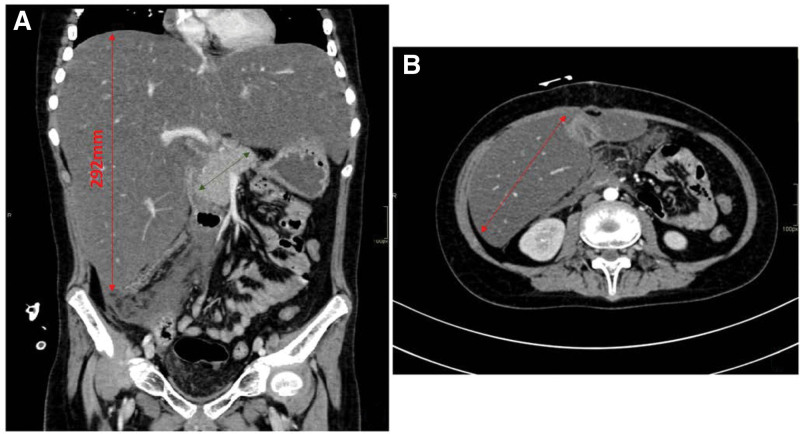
(A–B) Abdominal CT. Siemens Definition AS + spiral protocol before and after iv. admin-istration of a contrast agent. The liver was enlarged (dimension CC approx. 292 mm-red arrow), homogeneous, with clearly reduced density, without visible focal lesions. The gallbladder was partially constricted with signs of wall edema. In the lumen of the gallbladder there were 3 small gallstones 2–3.5 mm long. Pancreatic (green arrow) head was enlarged by dim. AP 29 mm with slightly blurred contours. The pancreatic parenchyma presented normal contrast enhancement (no areas of necrosis). Body and tail of pancreas were not enlarged, dim. AP 13 mm and 20 mm. Edema of adipose tissue and banded fluid areas were visible in the region of the head of the pancreas. In the area of the pancreas and along the mesentery of the intestine, there were moderately numerous lymph nodes with a diameter of in the short axis up to 10 mm. AP = acute pancreatitis, CT = computed tomography.
